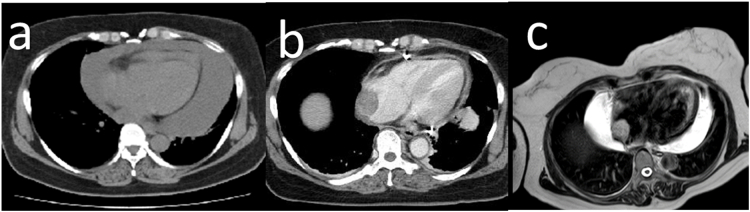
Computed tomography shows (a) large epicardial effusion and (b) tumor in the right atrium. (c) Magnetic resonance imaging shows epicardial effusion. The mass in the right atrium had an isointense to high-intensity signal on T2-weighted images.
